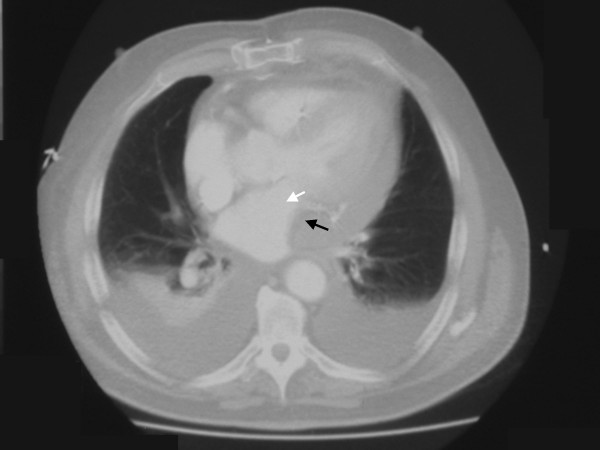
CT scan of the chest showing a large localised blood clot (black arrow) compressing the left ventricle (white arrow). Also note bilateral pleural effusions.
